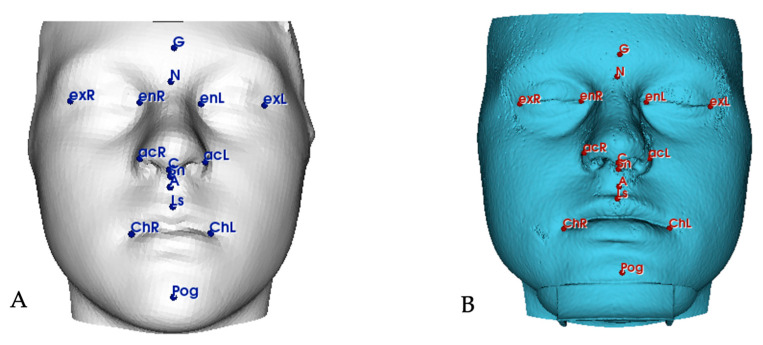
Automatic identification of 15 skin reference landmarks for automatic calculation of linear and angular measurements. (A) Scan performed with EM3D; (B) 3D model obtained from CBCT.
